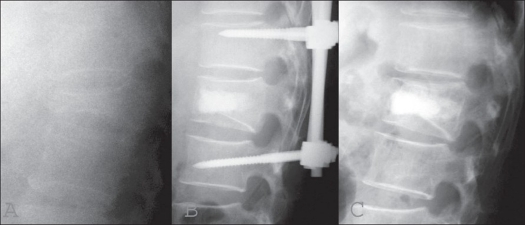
Lateral X-ray of thoracolumar spine of a 59-year-old man had a burst fracture of the first lumbar vertebra, with a neurological deficit, of ASIA Grade C on admission (Case 7). Lateral X-ray (A) A 59 years old man shows burst fracture of L1 vertebra. The patient was ASIA grade C on admission. The patient has vertebral kyphosis (T12-L2) 20 degrees. Immediate Post-operative lateral x-ray of dorso lumbar spine (B) shows post instrumentation anterior HA cylindrical stick and vertebral kyphosis corrected to 90. Standing lateral roentgenogram (C) taken two years after the operation showing re-establishment of sagittal alignment. Vertebral kyphosis had slightly deteriorated to 11 degrees. This patient had recovery of neurological loss of ASIA Grade from C to D
